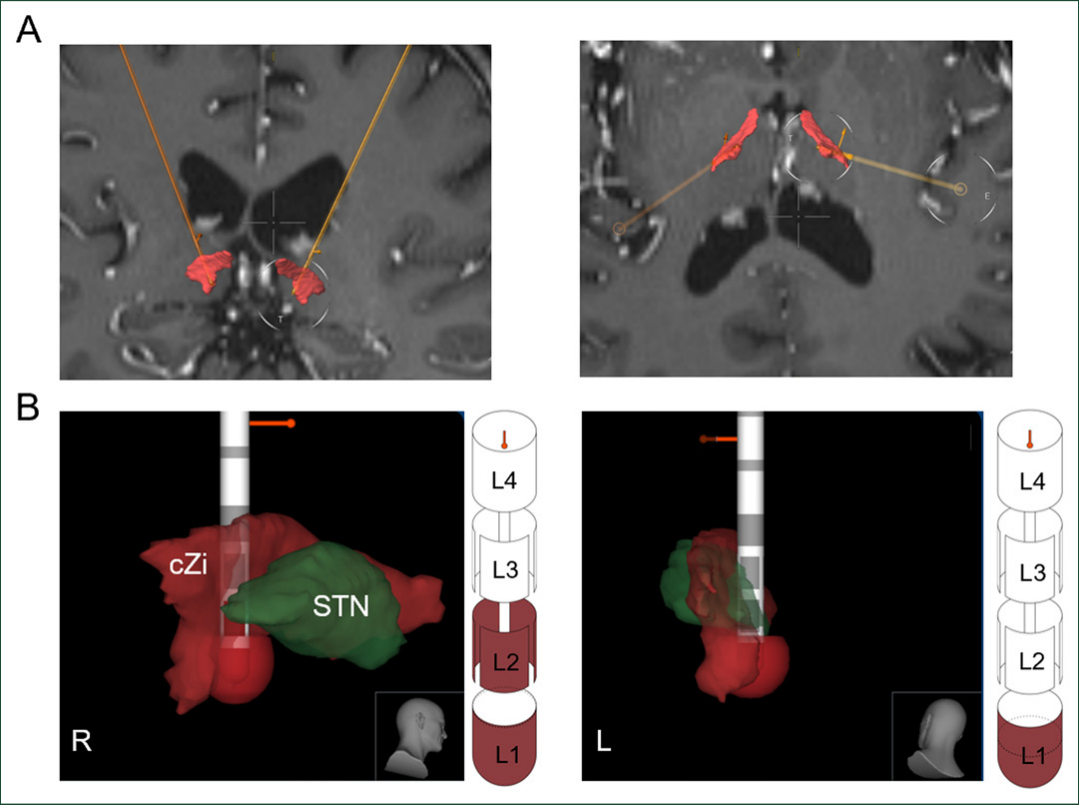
Reconstruction of DBS electrodes and programming. (A) Coronal and axial sections of the patient’s MRI overlayed with the placement of the right and left DBS leads in the cZi, indicated as the red region. (B) Programming of the leads targeting the cZi, with monopolar activation of the right lead consisting of 70% L1 and 30% L2 ring mode contact, and the left lead consisting of 100% L1 contact. cZi caudal Zona incerta, STN subthalamic nucleus.
